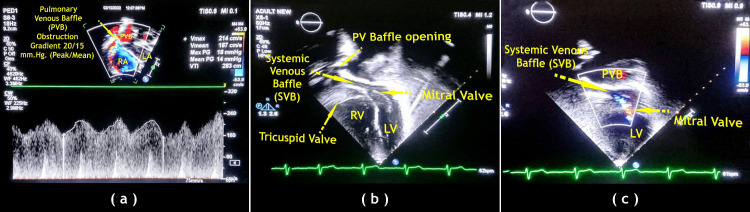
Post-operative 2D echocardiography images of modified Senning’s operation. (a) Case 1. (b) and (c) Case 2. Image Credits: Dr. Vishal V. Bhende.
